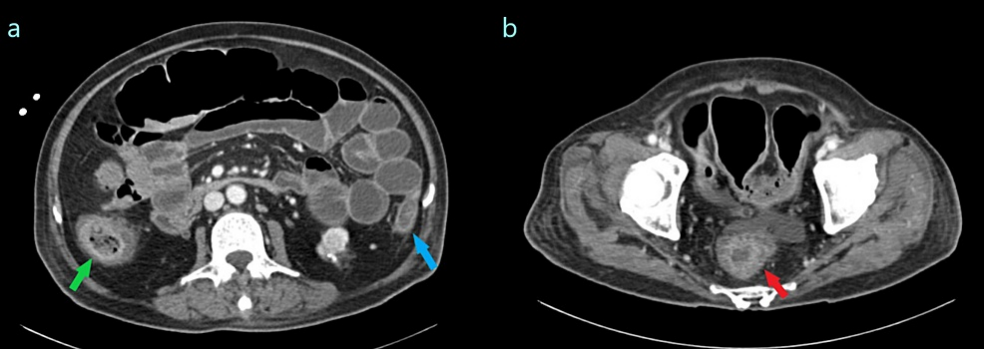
Computed tomography angiogram suggesting non-occlusive mesenteric ischemia. (a and b) Axial contrast-enhanced images through the abdomen demonstrate diffuse small bowel dilatation with colonic wall thickening predominantly affecting the watershed regions of the bowel despite patent vasculature. Green arrow: hepatic flexure. Blue arrow: splenic flexure. Red arrow: rectum.
